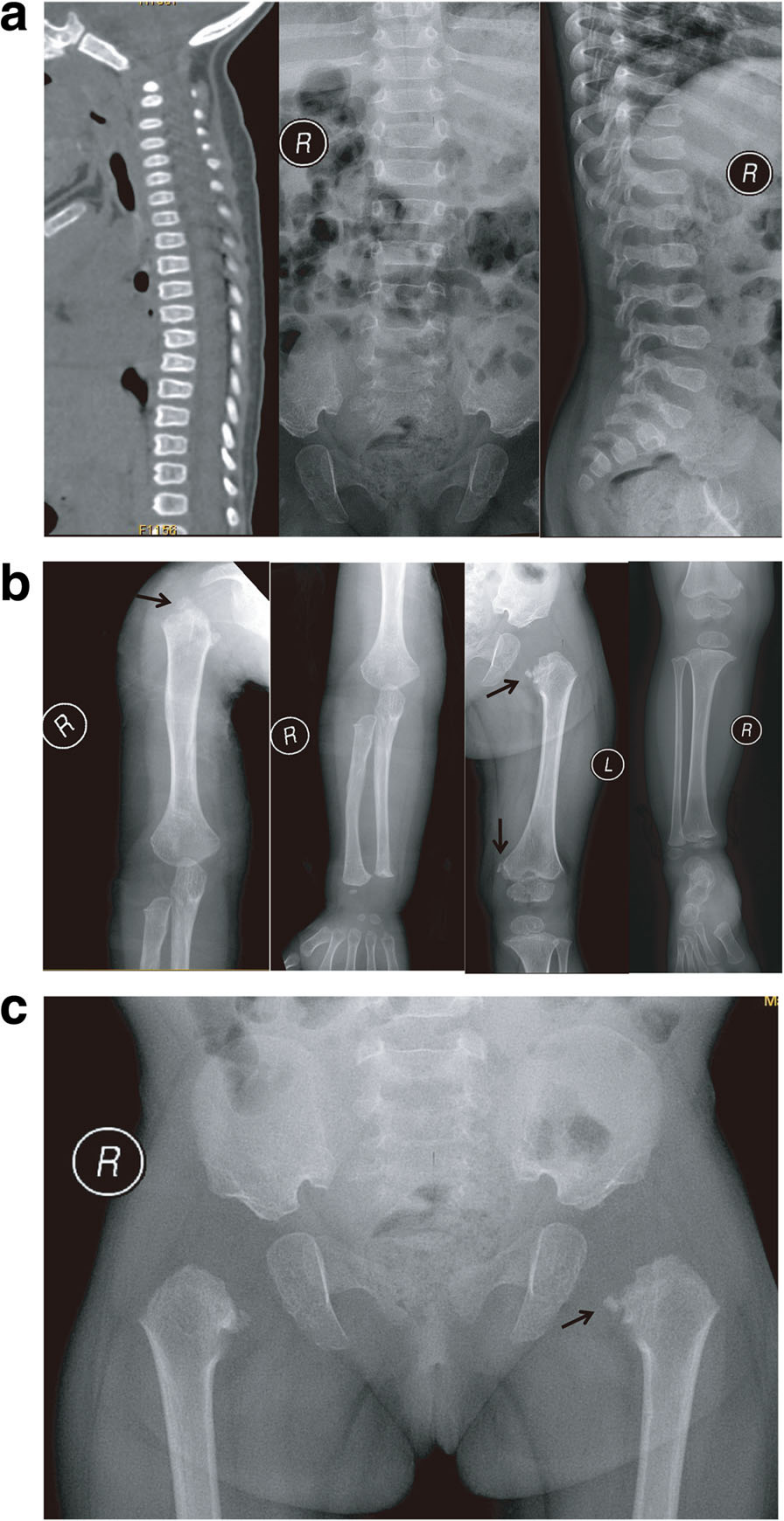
Radiographic findings of patient III-3. a Radiographic findings of the spine of patient III-3. The patient displayed platyspondyly (C3–C7), defects on the edge of the anterior vertebral bodies (L3–L5), and a slight shift of the vertebral axis. In addition, ovoid vertebral bodies, which are indicators of dysplasia, were observed in the CT images of the cervical spine. b Radiographic findings of the long bones of patient III-3. Bilateral humeri, ulnae, radii, femurs, and tibiofibulas were shortened. Bilateral femoral heads and necks, as well as the femoral head epiphyses and distal humeral epiphyses, were absent. The epiphyses of the upper humeri and distal tibias were dysplastic. The metaphyses of the proximal femurs and proximal humeri displayed a “dappling” appearance, resulting from the irregular intermingling of radiolucencies and radiodensities. The metaphyses in the proximal tibias were flared and irregular. Notably, “corner fracture” phenomena were observed in the right proximal humerus and bilateral femurs (arrows). c Radiographic findings of the pelvis of patient III-3. An irregular acetabular roof was observed
